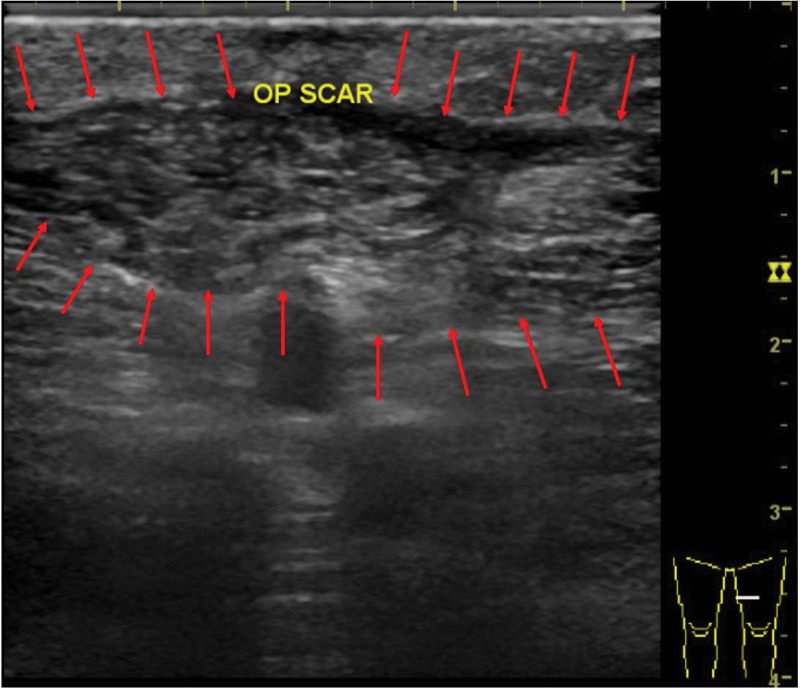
After follow-up 10 month, there was only scar at the operation site. No other residual parathyroid tissue seen in sonography.
